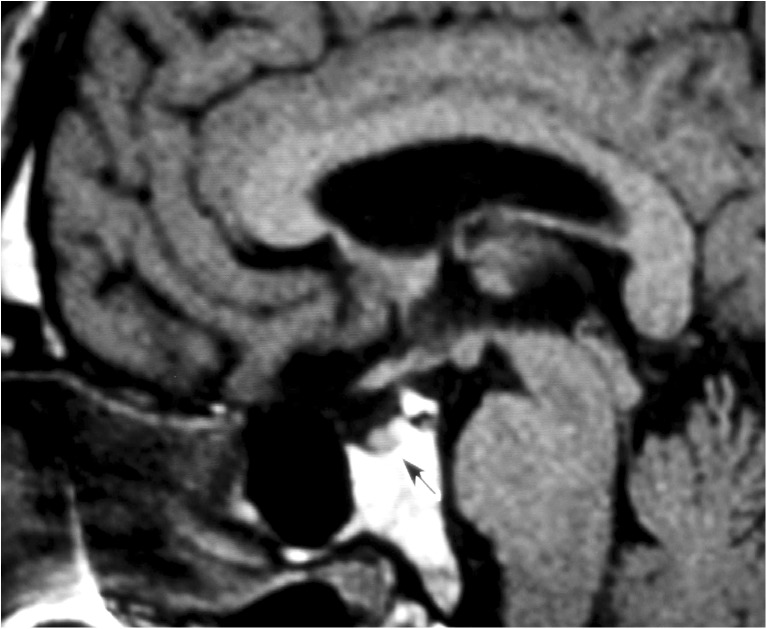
Midline sagittal MRI section showing hypoplasia of the anterior pituitary lobe in patient 2. The *arrow* indicates the small pituitary gland
